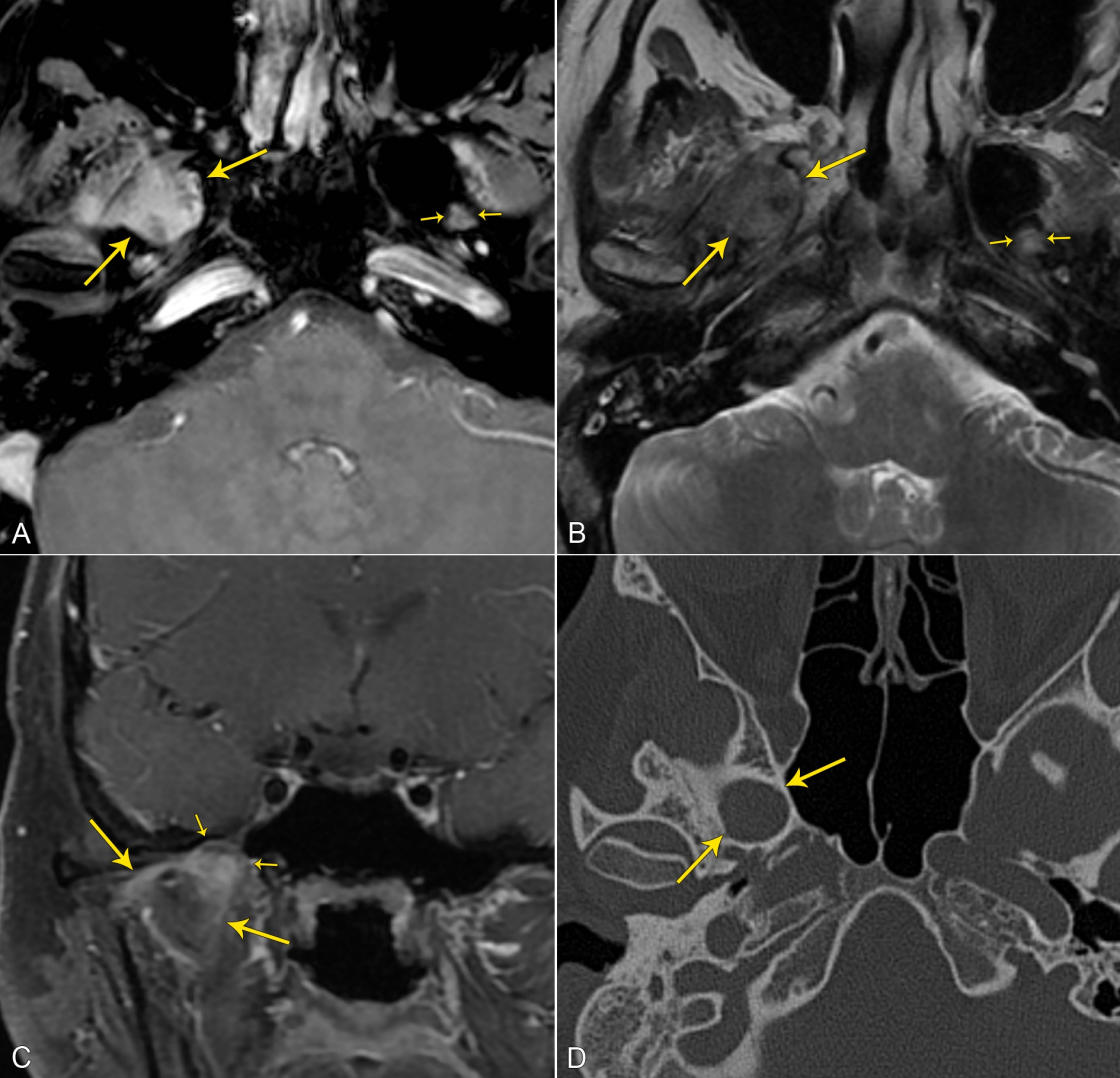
Preoperative Neuroimaging (A) Axial post-contrast fat-saturated T1-weighted MR image demonstrates a heterogeneously enhancing mass (long yellow-arrows) in the expanded right foramen of ovale. The normal left foramen of ovale is small by comparison (small yellow-arrows). (B) Axial T2-weighted MR image demonstrates the mildly heterogeneous mass (long yellow-arrows), predominantly isointense to gray matter, in the right foramen of ovale. The normal left foramen of ovale is small by comparison (small yellow-arrows). (C) Coronal post-contrast fat-saturated T1-weighted MR image demonstrates thin areas of linear enhancement (long yellow-arrows) extending into/infiltrating the muscles of mastication from the mass in the foramen ovale (short yellow-arrows). (D) Axial bone-window CT of the skull base demonstrates smooth, non-destructive expansion of the right foramen of ovale.
